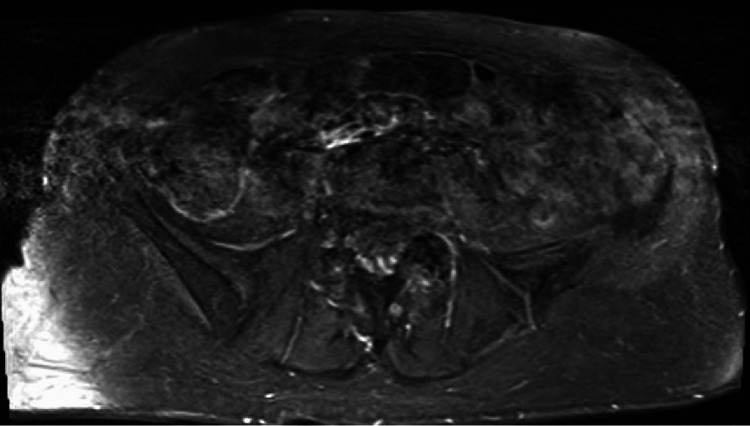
Lumbosacral plexus MRI (T1 fat sat transverse view) unremarkable for any evidence metastatic invasion.
